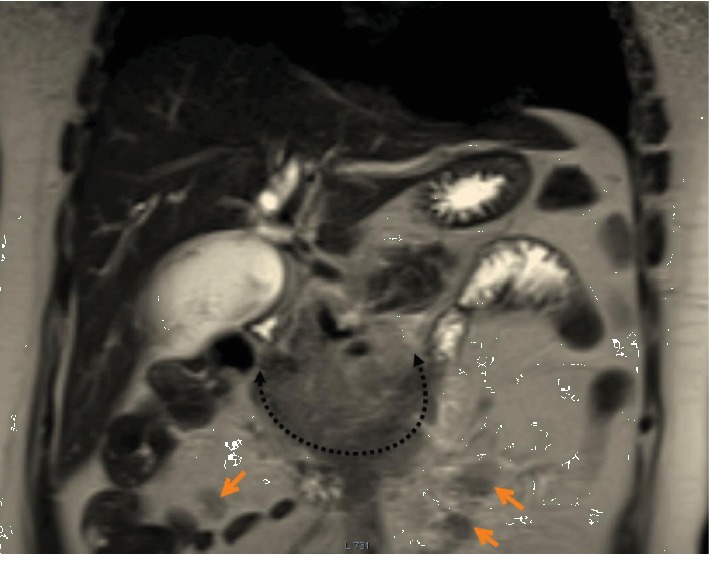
Magnetic resonance imaging (MRI) of the abdomen. Coronal slice shows the uncinate process mass (dotted black arrow) and multiple neoplastic peripancreatic lymph nodes (orange arrows).
